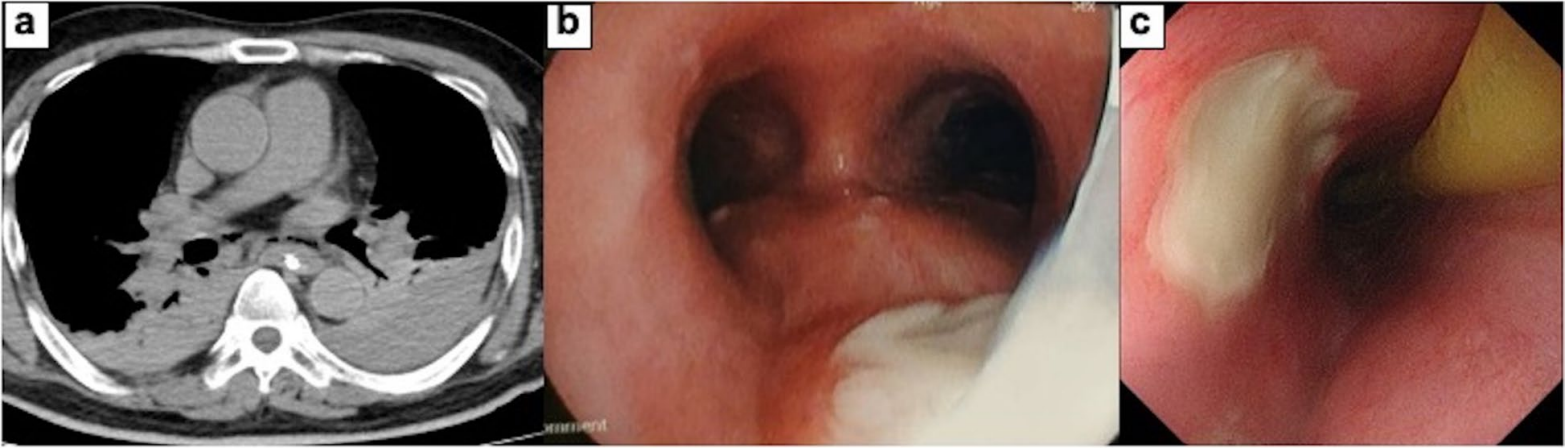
a CT revealed that mediastinal emphysema and pleural effusion in the left thoracic cavity had worsened on hospital day 2. b An ulcerative lesion above the tracheal bifurcation was observed on bronchoscopy. c EGD revealed ulcerative lesions with a size of 10 mm on the middle thoracic esophagus. EGD, esophagogastroduodenoscopy
